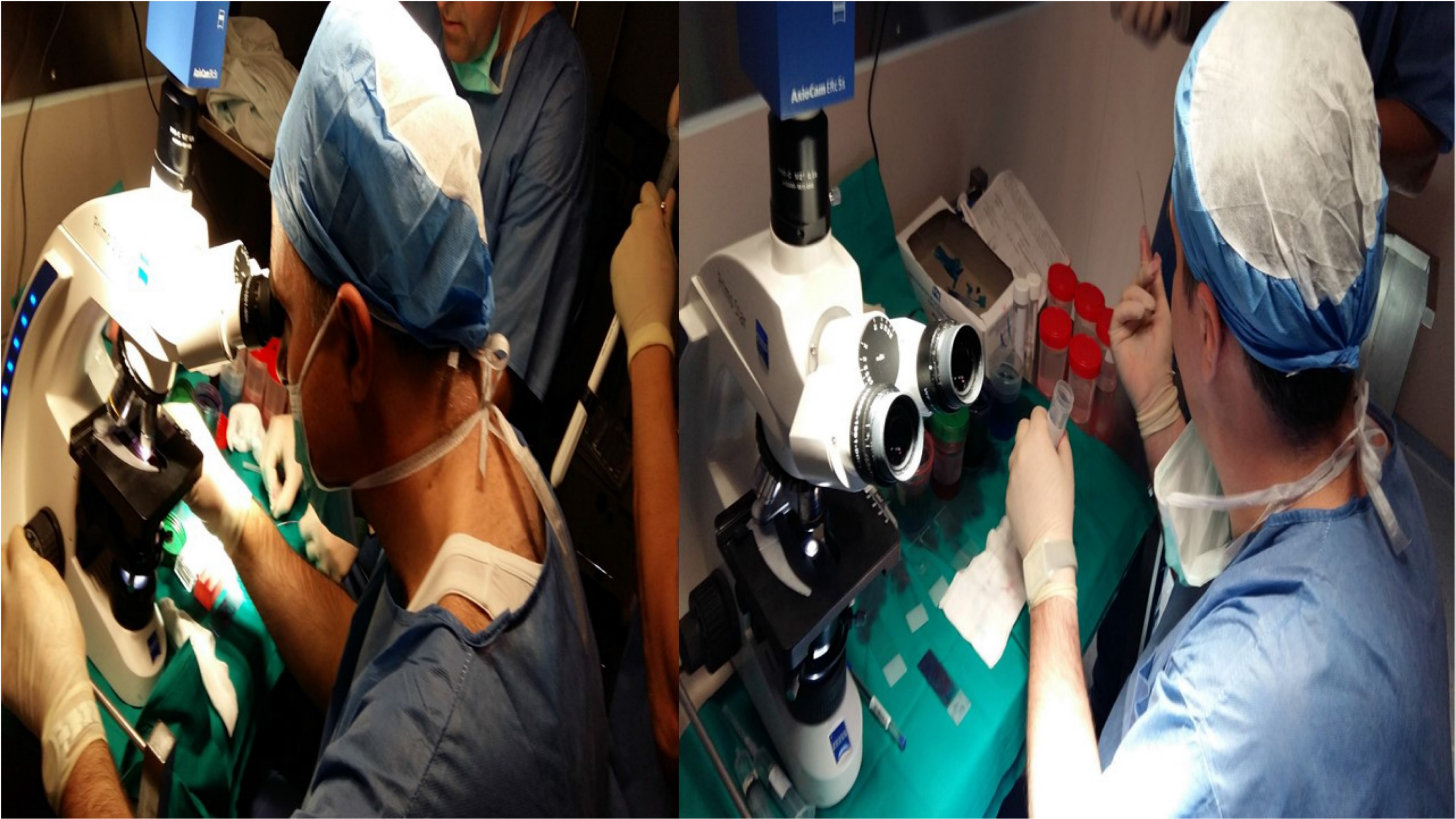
Rapid on site evaluation from convex endobronchial ultrasound with 22G fine needle aspiration biopsy (Mediglobe needle).
